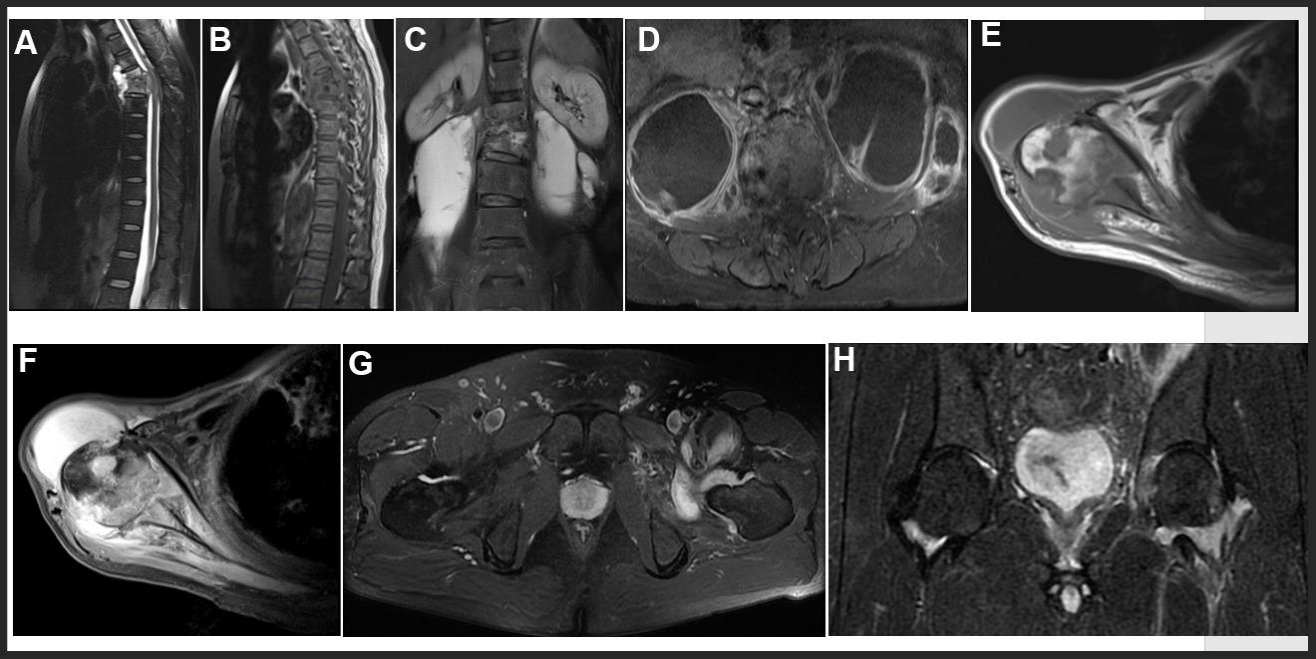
Representative examples of MRI in OTB patients. (A, B) Thoracic spine tuberculosis: showing destruction of vertebral and paravertebral abscess. (C, D) Lumbar spine tuberculosis: showing destruction of vertebral and psoas abscess. (E, F) Right shoulder tuberculosis: showing worm like destruction of the right humeral head with effusion in the articular cavity. (G, H) Left hip joint tuberculosis: showing left hip joint synovitis and synovial fluid.
